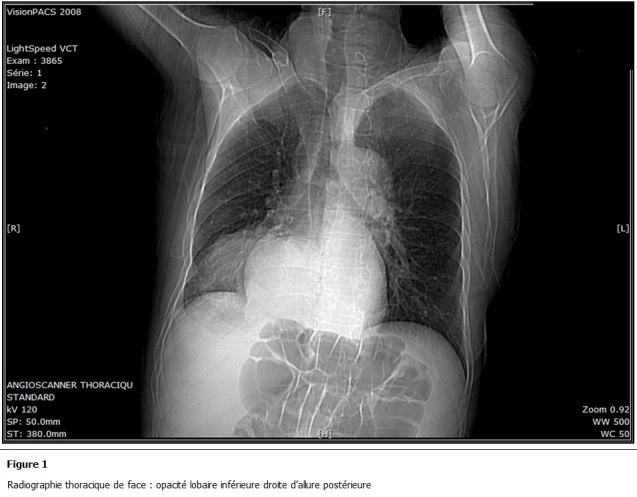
Radiographie thoracique de face: opacité lobaire inférieure droite d’allure postérieure
